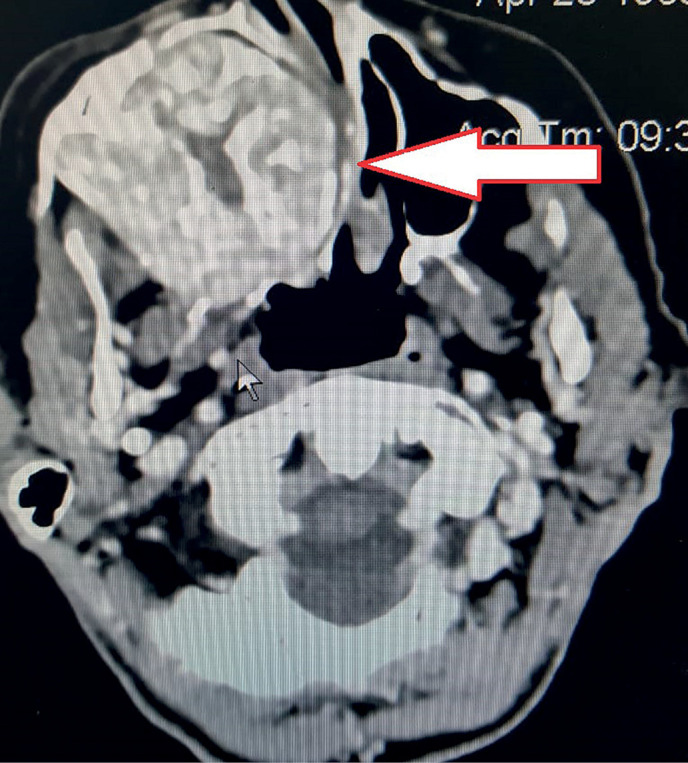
Figure 1. Computed tomography of the facial skull: the arrow shows the mass lesion of the maxillary sinus with spreading to the sphenoid sinus.
